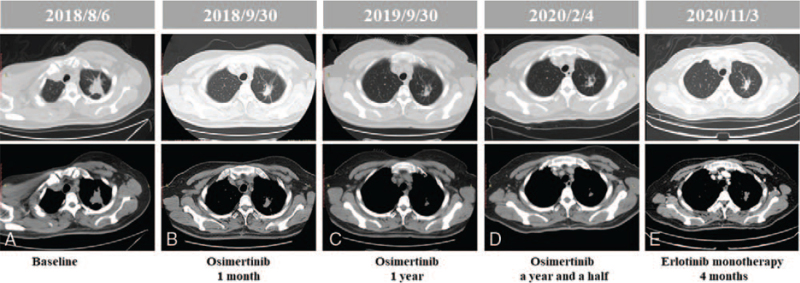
Computed tomography (CT) scan showed the changes of lung lesions during treatment. (A) Before treatment. (B) One month after osimertinib treatment, the tumor shranked. (C) One year after osimertinib treatment, the tumor shranked. (D) A year and a half after osimertinib treatment, the tumor was as before. (E) Four months after erlotinib monotherapy, the tumor had little change.
